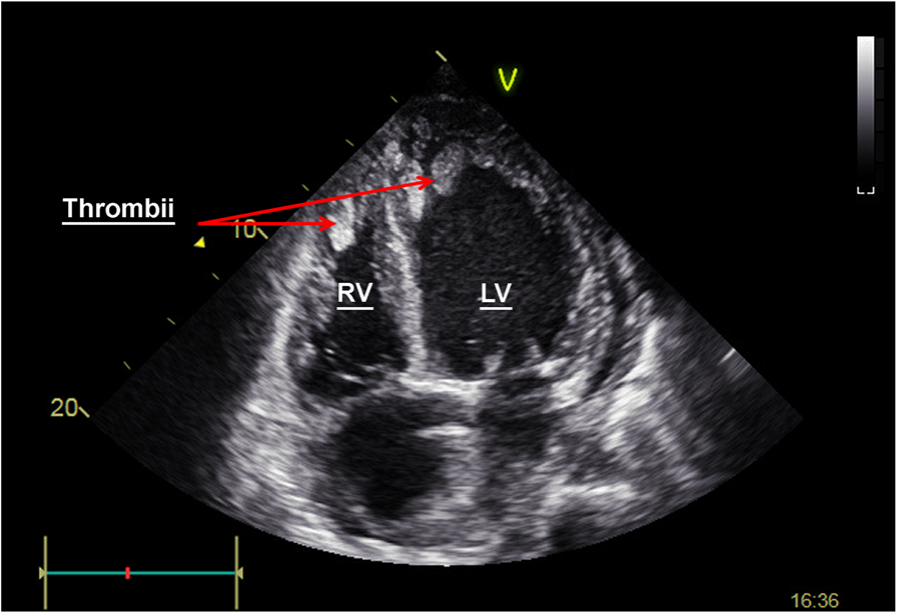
Trombus formation in left and right ventricle in echocardiography. RVOT - right ventricular outflow tract,, LV – left ventricle
